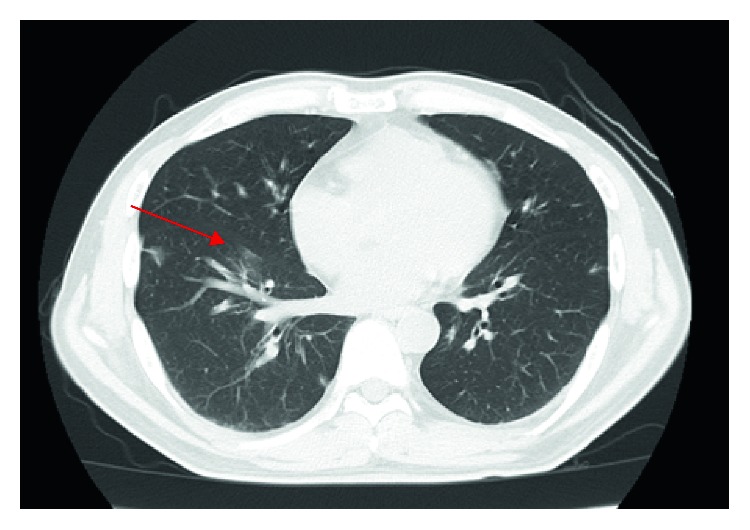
The chest computed tomography on the 38th day showing multiple nodules disappeared (red arrow).
